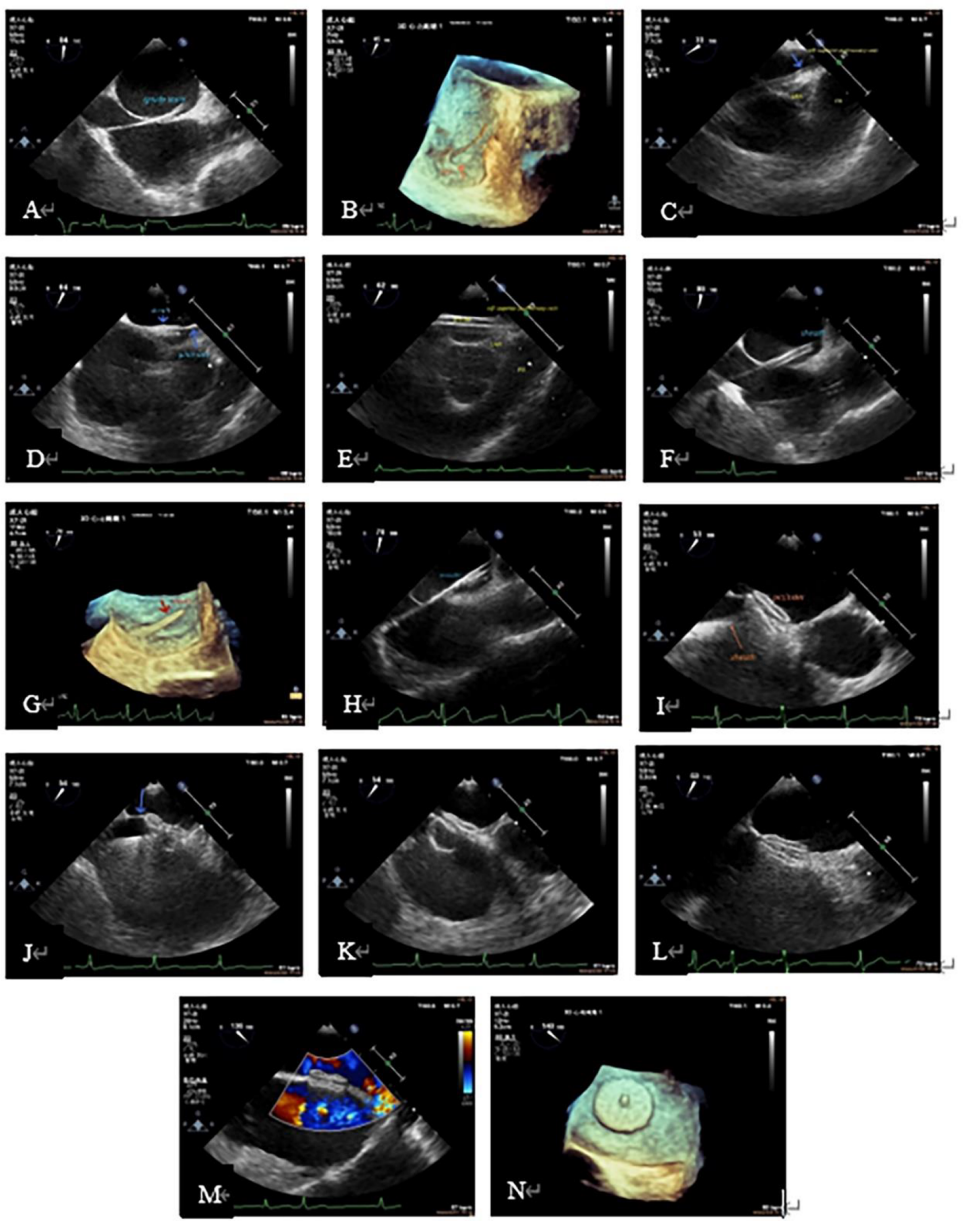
Procedure demonstration during TEE-guided percutaneous PFO closure. (A): Guide wire through PFO tunnel; (B): 3D visualization of the guidewire reaching the LA through the PFO. (C): Guidewire leading to LSPV (blue arrow); (D): the sheath (short blue arrow) passed through the PFO into the LA via the guide wire (long blue arrow). (E): Sheath entering the LSPV; (F): the sheath in the LA; (G): three-dimensional image showing the delivery sheath reaching the LA via the PFO; (H): occlude in the delivery sheath (echogenic part in the sheath); (I): after completely releasing of the two discs of the occlude, the atrial septum was located between the two discs, when the delivery sheath was still connected to the right atrial disc (arrow). (J): With the help of the sheath tube, the occlude was “pushed” to the LA, and the primary septum was pushed up (arrow). (K): The whole occlude was “pulled” to the RA, and the slight deformation of the right atrial disc could be seen. (L): State after the complete release of the occlude (complete detachment of the sheath from the right atrial disc). (M): Color Doppler showed no residual shunt. (N): Left atrial disc showed in 3D. 3D, three-dimensional; LA, left atrium; LSPV, left superior pulmonary vein; PFO, patent foramen ovale; TEE, transesophageal echocardiography.
